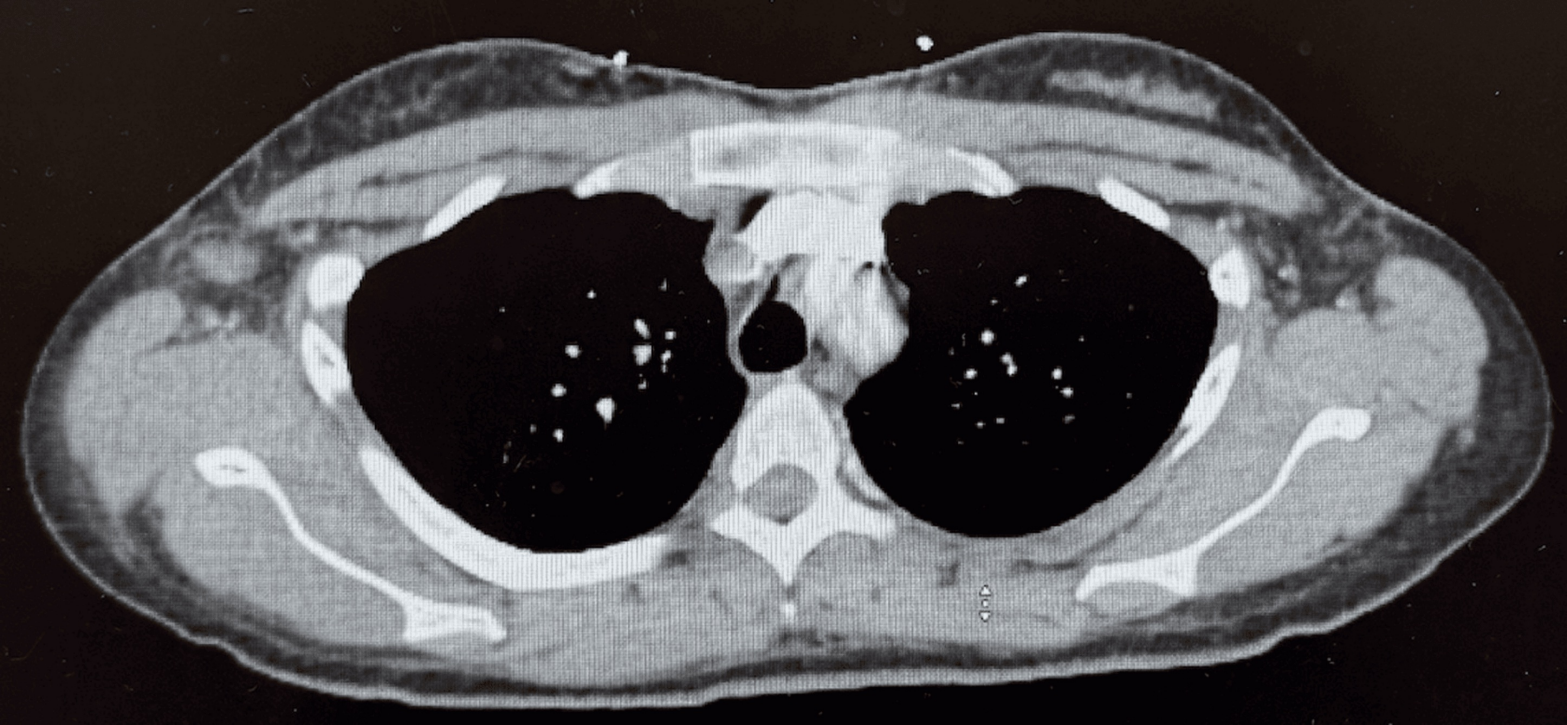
CT of the chest displaying no pathological findings, including consolidations, pleural effusions, interstitial infiltrates, or abscesses, supporting the notion that the source of infection is not pulmonary. CT: computed tomography
